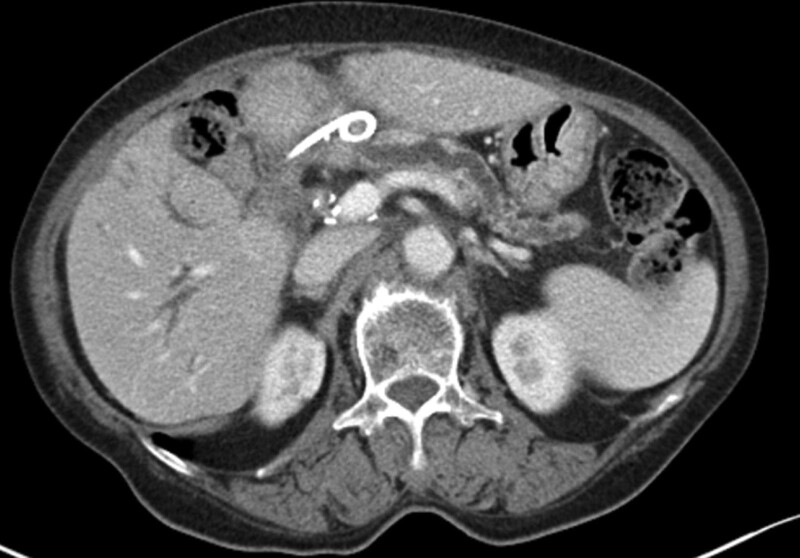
Follow-up abdominal computed tomography 3 weeks after the endoscopic treatment showed a plastic stent and resolution of the pancreatic pseudocyst.
